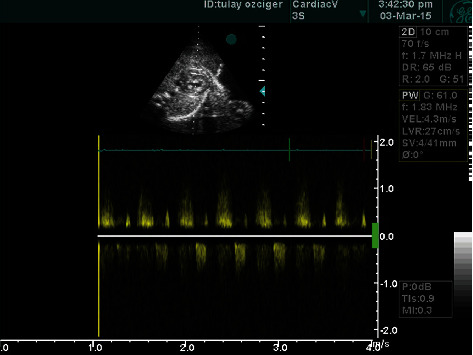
Pulsed Doppler examination of VCAC demonstrating bidirectional flow.
